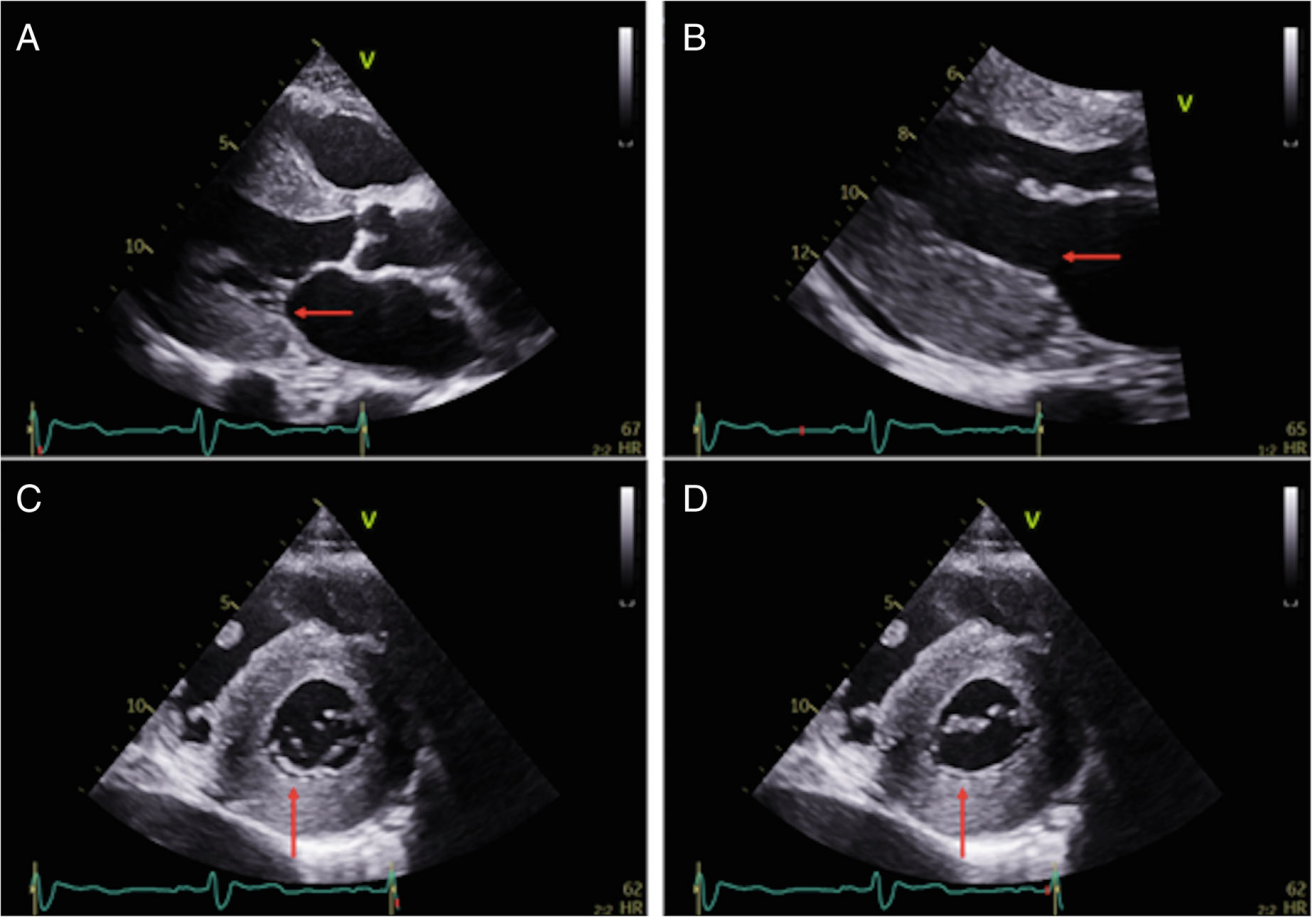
Transthoracic echocardiogram in a patient with transthyretin amyloid cardiomyopathy: mitral valve. A 78‐year‐old lady with V122I‐associated hereditary transthyretin amyloidosis. (*A*) Two‐dimensional transthoracic echocardiographic parasternal long‐axis view of the mitral valve with both thickened and markedly shortened posterior mitral leaflet (red arrow). (B) Zoomed parasternal long‐axis view of the mitral valve in diastole where the posterior mitral leaflet appears to be completely ‘disappeared’ (red arrow). (*C*) Short‐axis view of the mitral valve in systole, confirming a short posterior mitral leaflet (red arrow) in early systole with eccentric posterior coaptation line. (*D*) Short‐axis view of the mitral valve in diastole, demonstrating the ‘disappeared’ posterior mitral leaflet as fixed and englobed into the posterior wall (red arrow).
